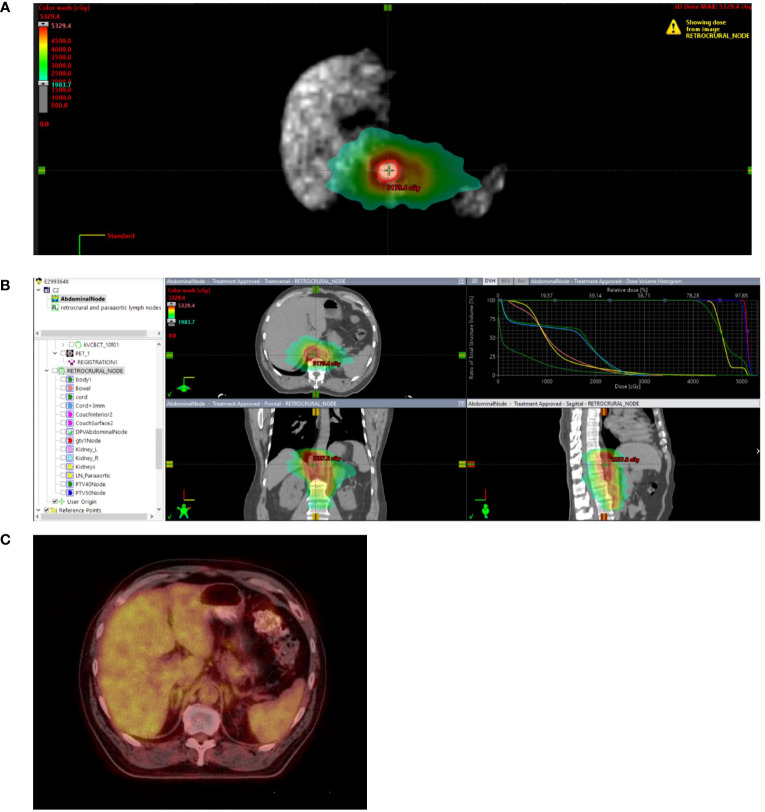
Stage IIIB rectal cancer initially treated with total neoadjuvant therapy followed by sphincter-sparing surgery with pathologic complete response followed by 2 additional cycles of adjuvant FOLFOX. While on surveillance, the patient presented with an elevated CEA of 18.3. PET/CT demonstrated a new 3.5 cm retrocrural node with a SUVmax of 3.5 without additional areas of FDG avid disease. Biopsy confirmed metastatic rectal adenocarcinoma. (A) Treated with involved site radiotherapy to 50 Gy in 10 fractions to the PET positive node while covering PET negative prominent paraaortic nodes to 40 Gy in 10 fractions. (B) Radiation plan demonstrating selective sparing of uninvolved bowel, liver, kidneys and spinal cord. (C) Restaging 6 month PET/CT negative. Remains on surveillance off therapy more than 3 years after treatment with a recent CEA 1.3, undetectable circulating tumor DNA and negative CT and MRI.
